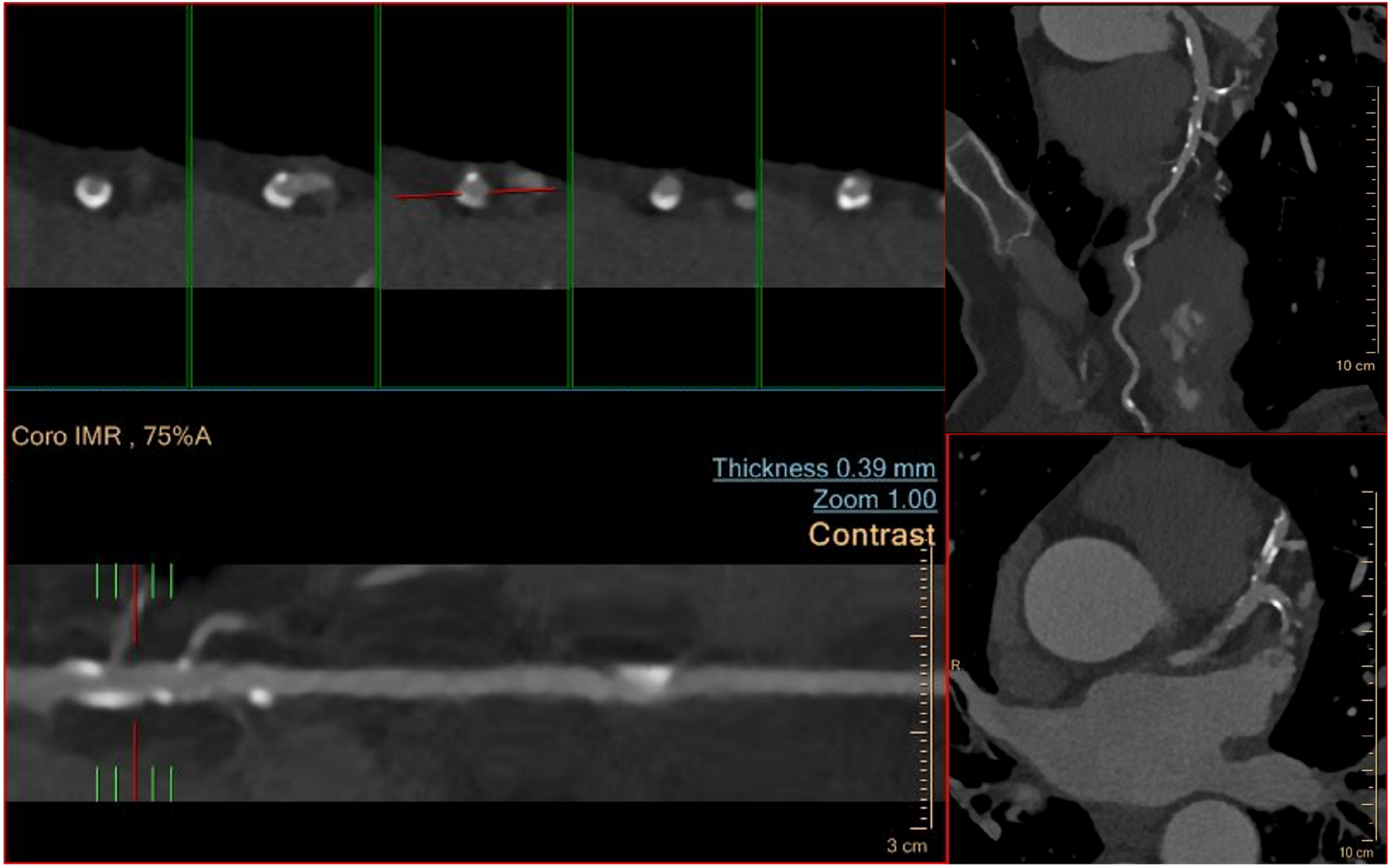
Example of CT angiography. In this example, multiple calcified plaques can be appreciated.
